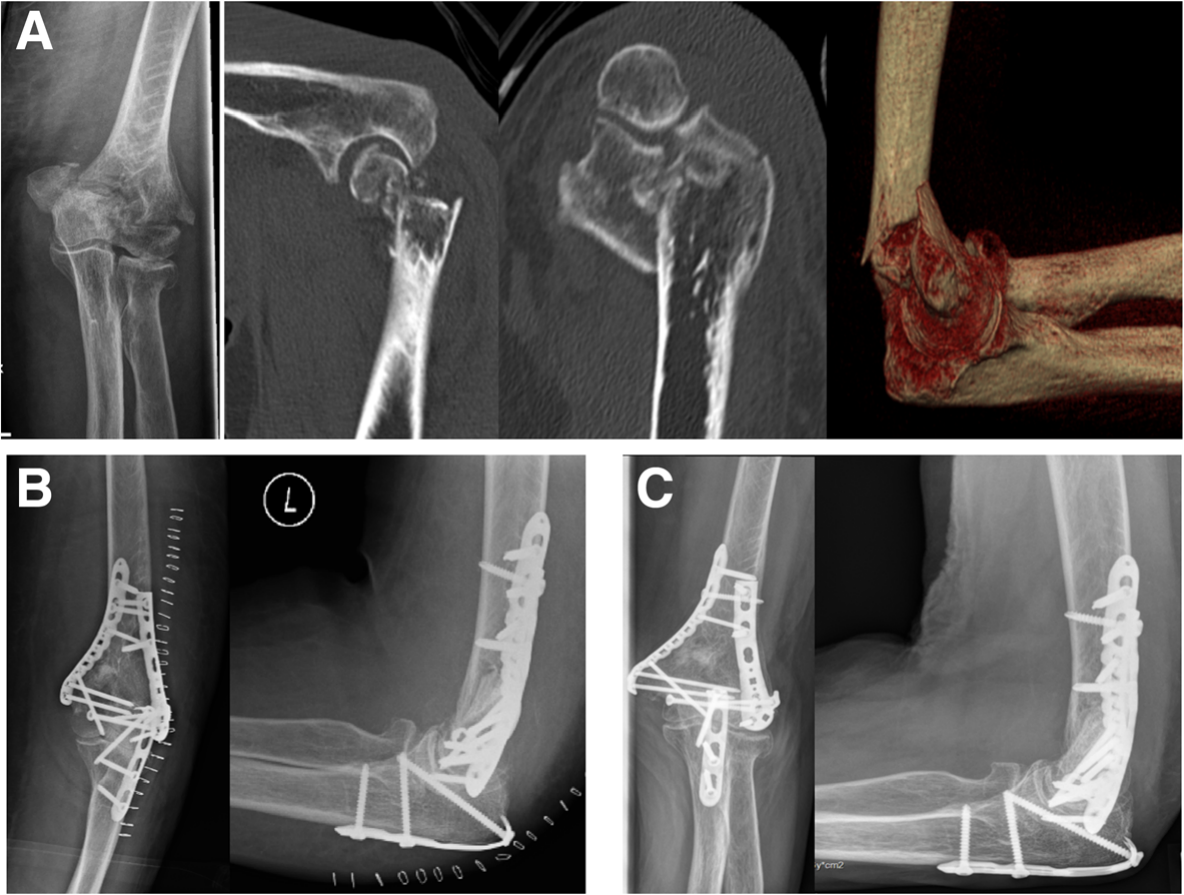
ORIF of a distal humerus fracture (AO 13-C3) with the DePuy Synthes® VA-LCP System. Radiographs and CT Scans of a 82-year-old female patient with a AO type 13-C3 fracture resulting from a fall. Preoperative x-rays and CT Scans (a), postoperative results, 6 weeks (b) and 12 months (c) following DePuy Synthes® VA-LCP plating
